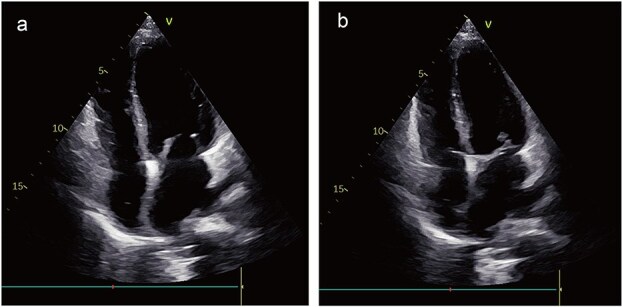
Postoperative images. The echocardiogram indicated no signs of local recurrence (a) and (b).
